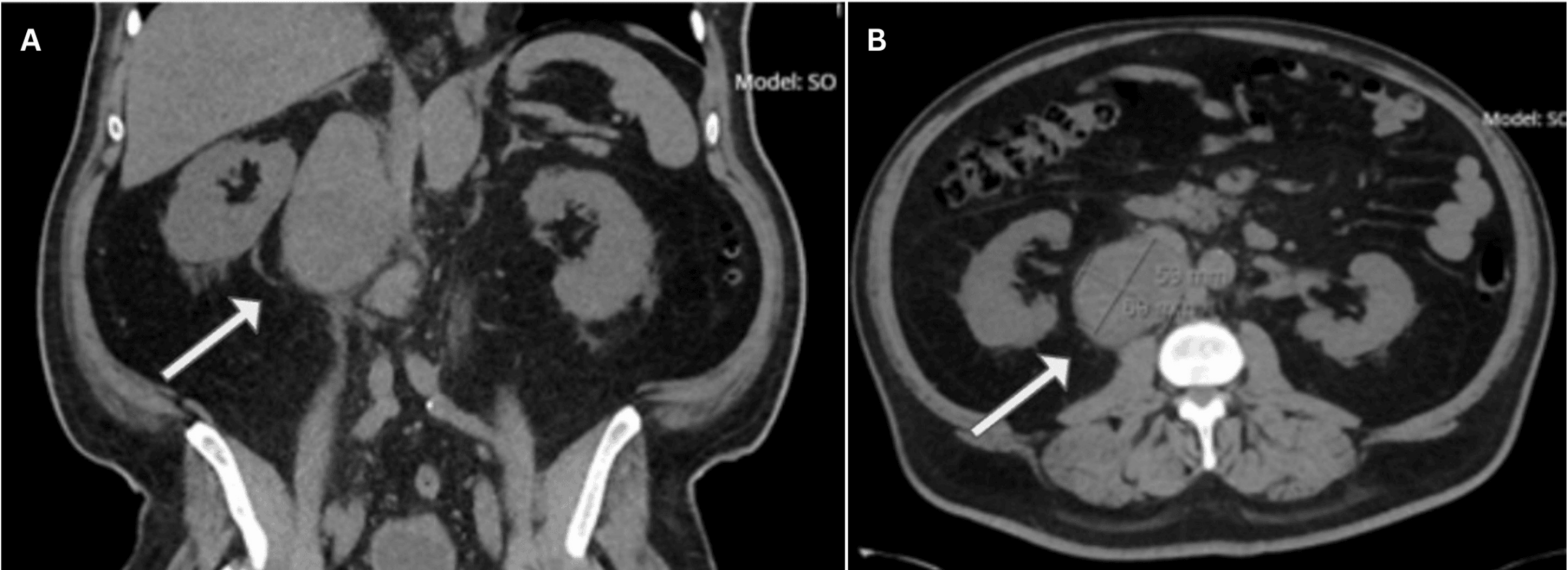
(A, B) CT-guided core needle biopsy confirmed the retroperitoneal mass as RCC (white arrows) CT: computed tomography, RCC: renal cell carcinoma
